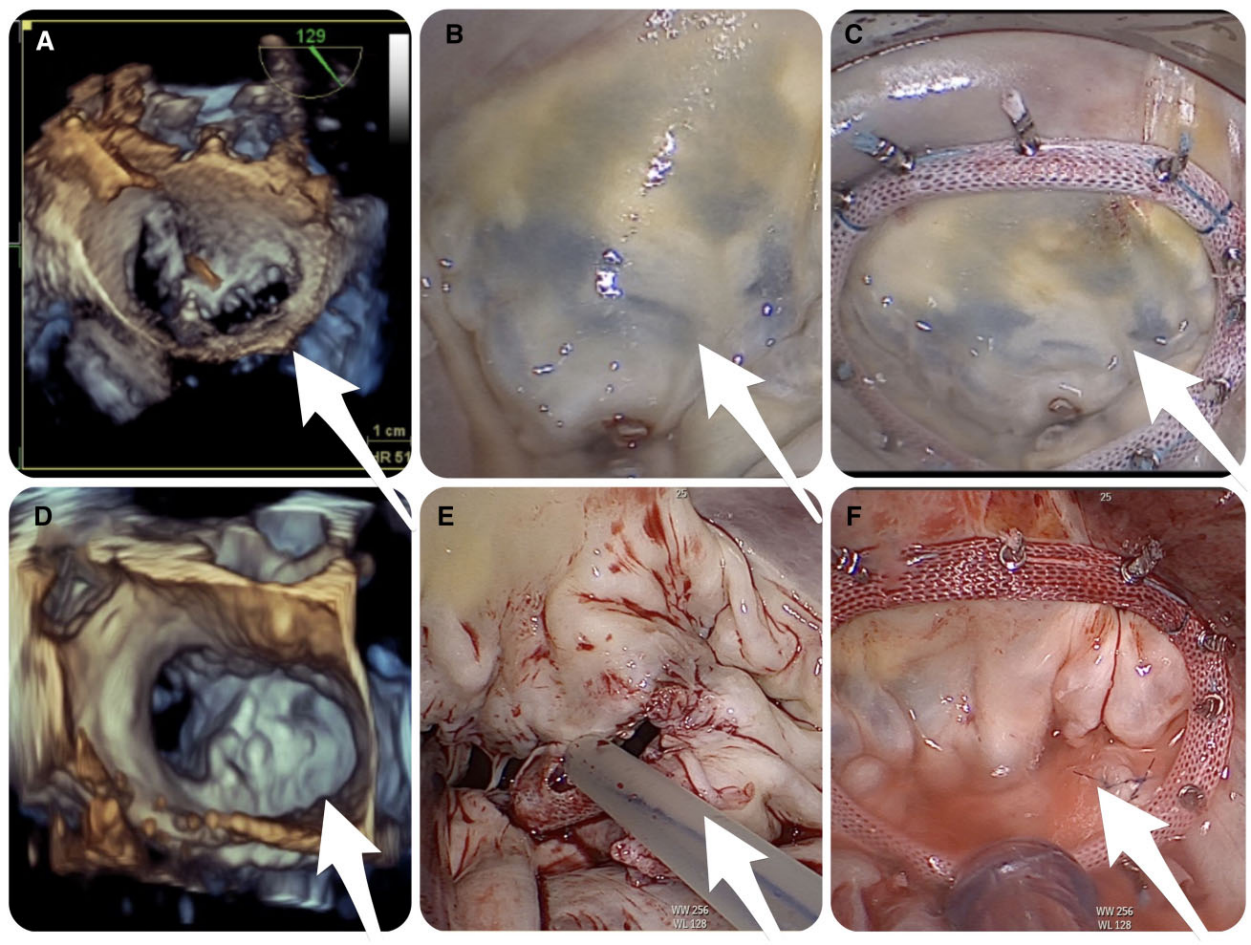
(A) A MitraClip device in the correct position and annular dilatation. (B) A saline test shows no leaflet prolapse, but regurgitation at the P1 and P2. (C) The clip was kept in situ and the annulus was supported with ring annuloplasty. (D) Perioperative transoesophageal echocardiography showing 2 MitraClip devices. (E, F) The surgical removal of the MitraClip device to maintain valvular integrity, facilitating subsequent neochordae implants, cleft closure and ring annuloplasty.
